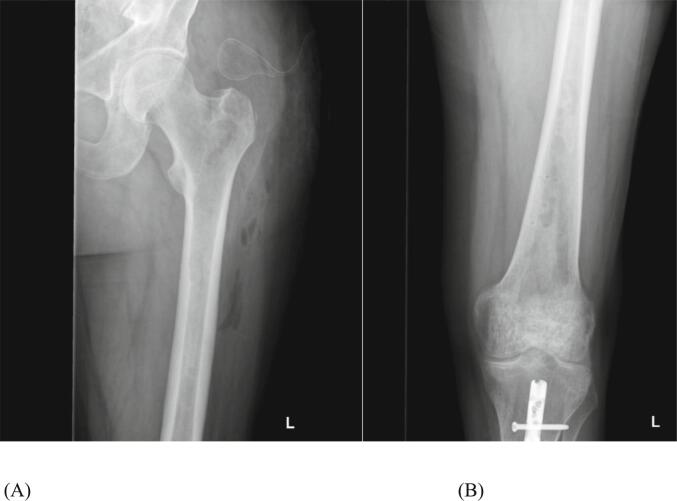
Postoperative radiographs after revision surgery demonstrating complete removal of metal debris at the proximal (A) and distal (B) femur.
